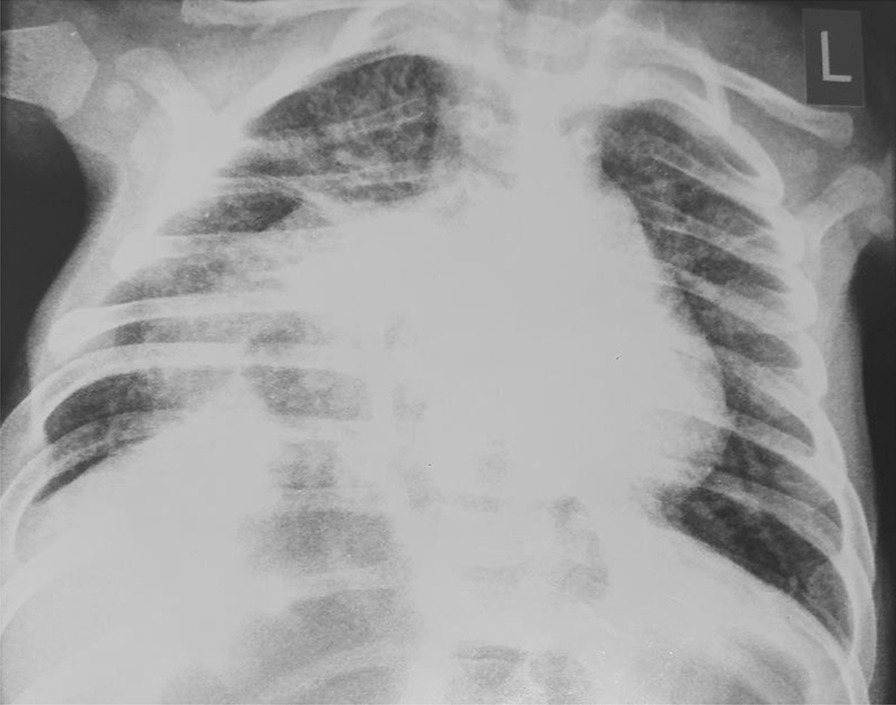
Before the management of bacterial pneumonia, chest radiography shows an elevation of right hemidiaphragm at the medial part and right lung infiltration in the index baby
